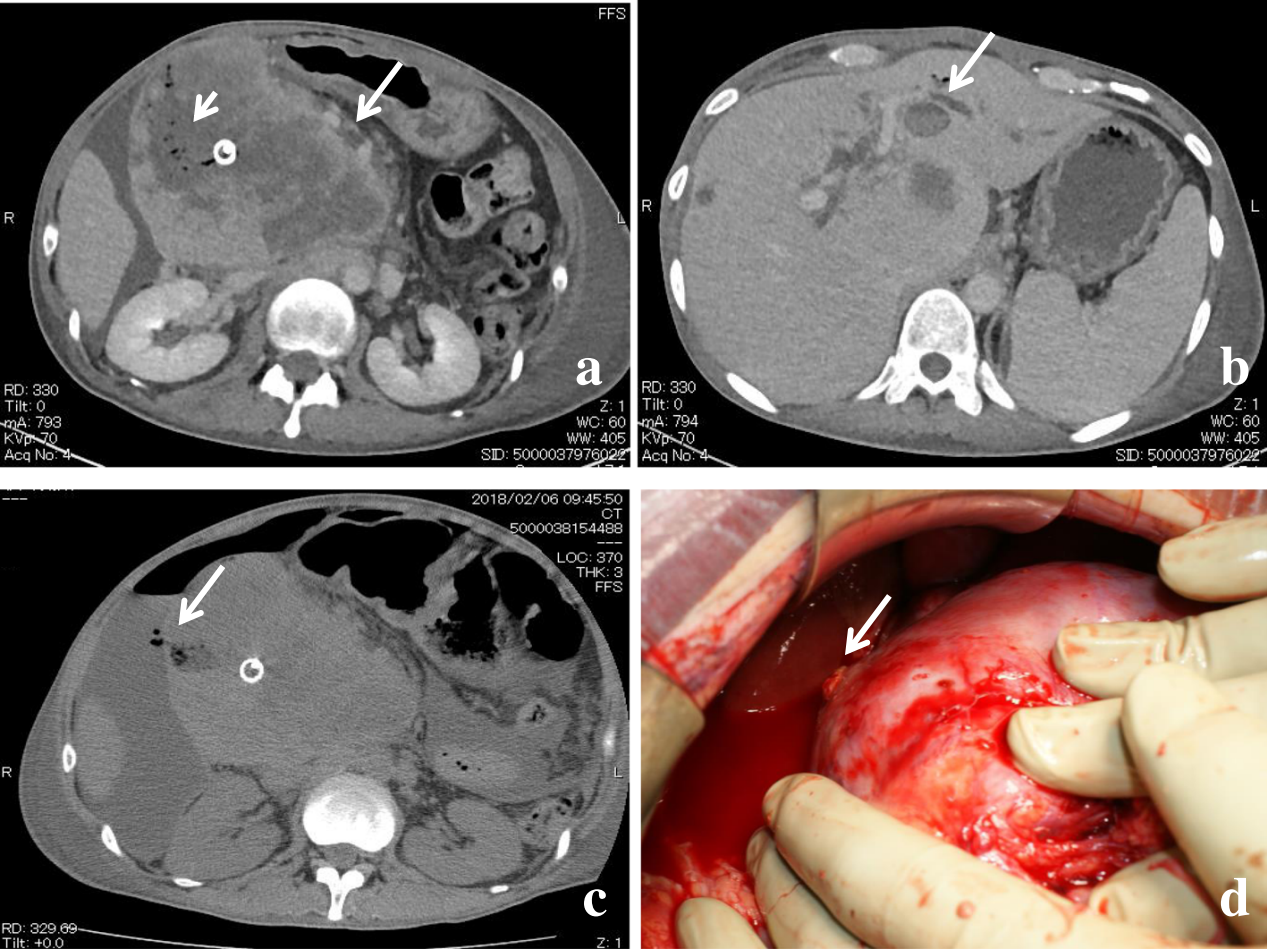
a Dynamic CT after 5 cycles of chemotherapy. Lymph node metastasis spread (maximum diameter, 120 mm) (arrow). The duodenum was strongly depressed, and air was observed in the tumor (short arrow). b The size of the intraductal tumor at B2 did not change after the patient received chemotherapy (arrow). c Abdominal CT revealed ascites and continuous free air inside the abdominal tumor as lymph node metastasis in the hepatoduodenal ligament (arrow). d Intraoperative macroscopic findings. The ruptured tumor was observed in the hepatoduodenal ligament (arrow)
